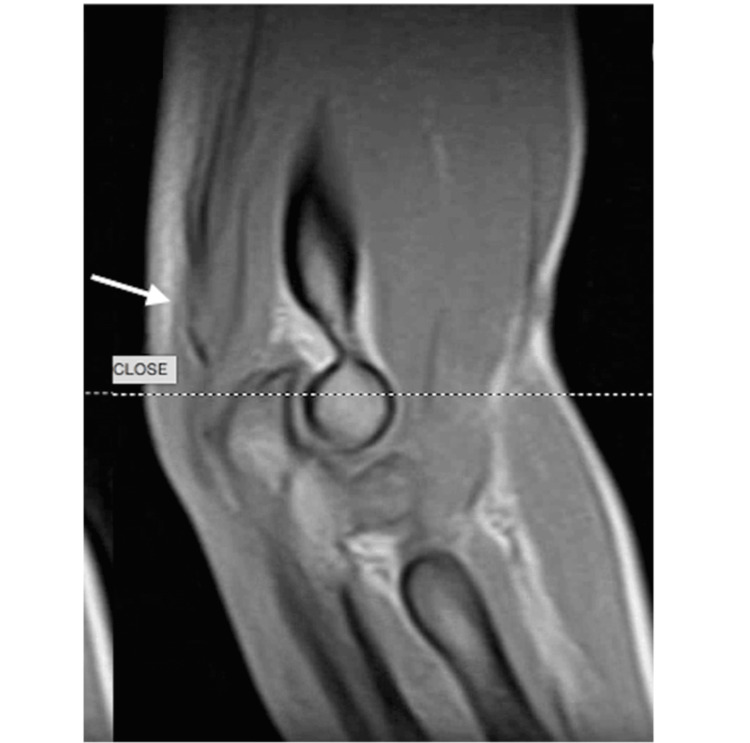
MRI showing a full-thickness triceps tendon tear with triceps muscle contracture
